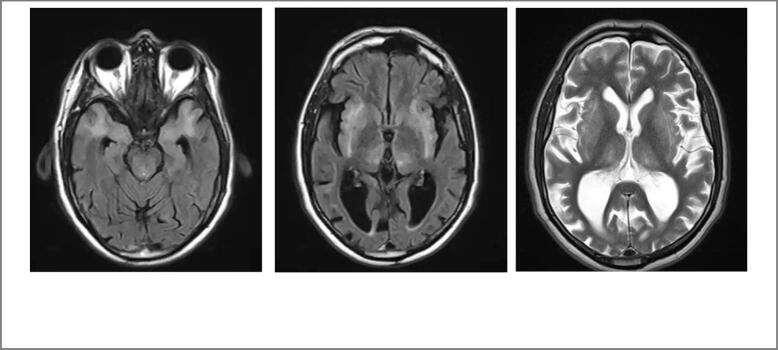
Post-treatment MRI shows a slight resolution of lead deposits.
